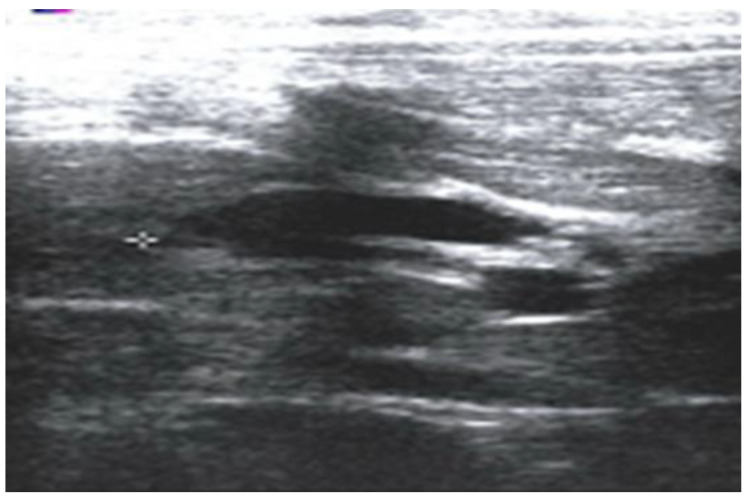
An abdominal ultrasound of a 2-year-old girl shows a dilated CBD with a diameter of 8.2 mm.
